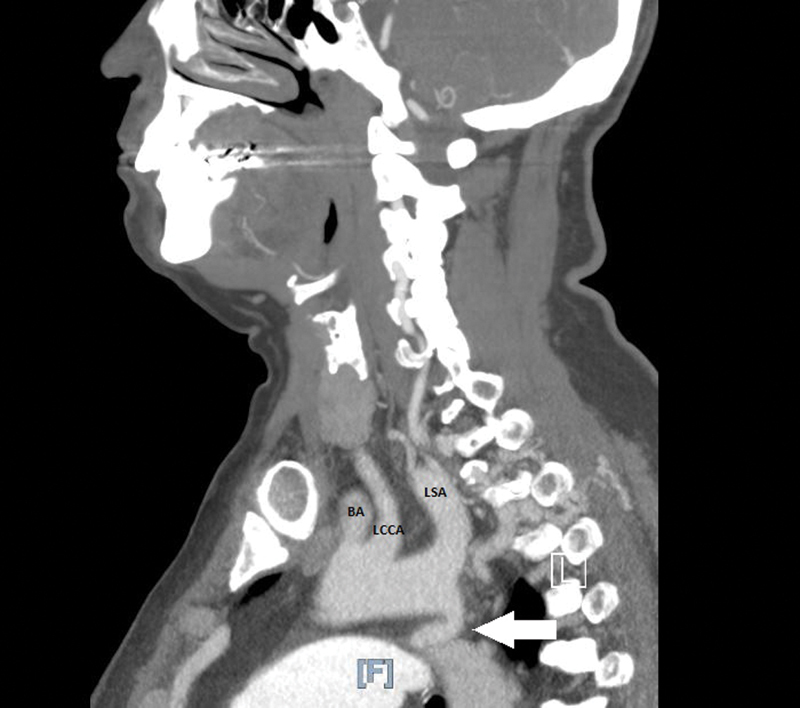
Computed tomography angiography notable for aortic coarctation with narrowing of aortic lumen distal to origin of left subclavian artery. White arrow pointing at site of coarctation. BA, brachiocephalic artery; LCCA, left common carotid artery; LSA, left subclavian artery.
